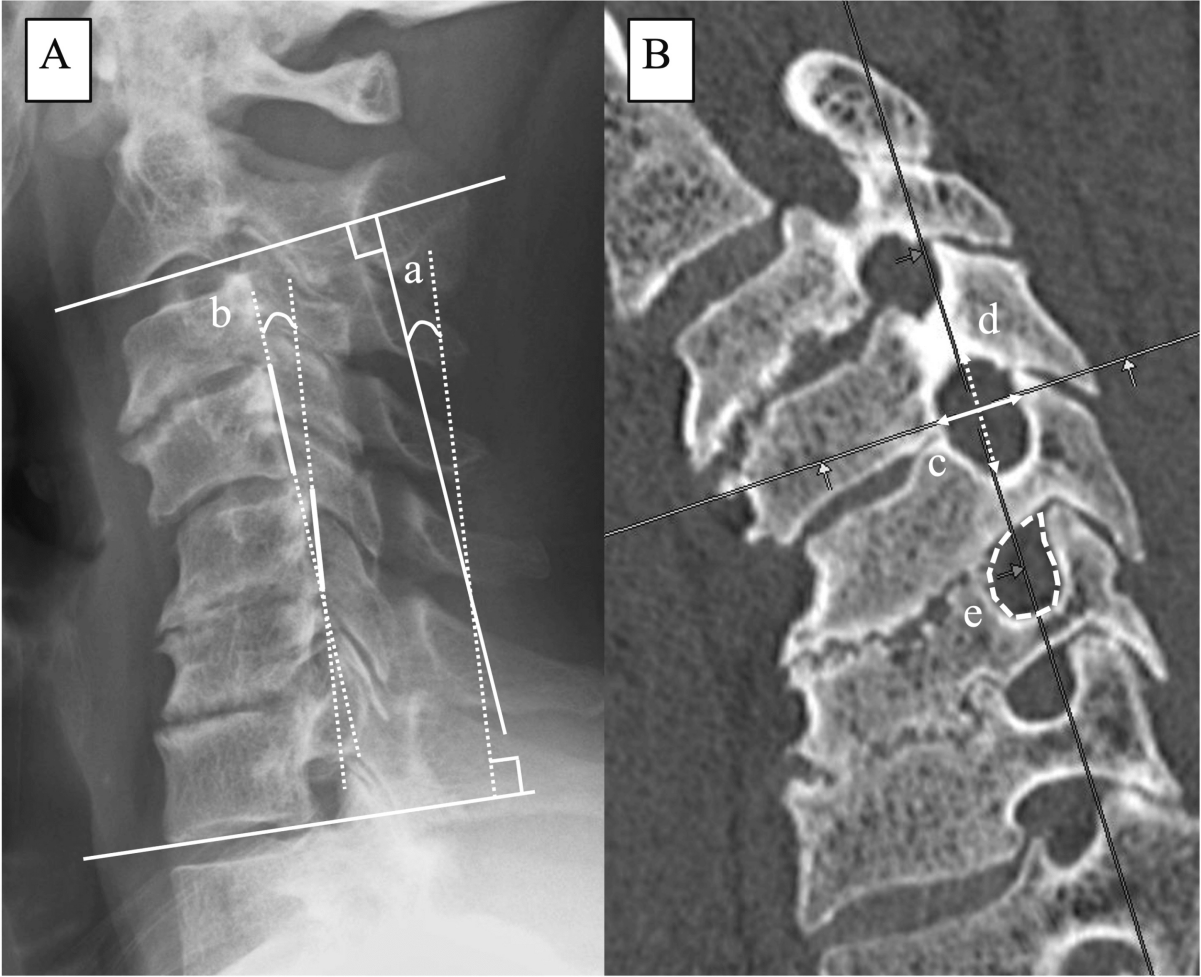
Radiological measurements and 3d-CT images. (A)  C2‒7 angle (a) and local segmental angle (b) were measured on a lateral radiograph in the neutral position. The segmental local angle (SA) was determined as the line from the posterior margins of the superior and inferior vertebrae to each targeted intervertebral foramen. (B) Sagittal CT image reconstructed using MPR.  c: The anteroposterior diameter (AP), d: craniocaudal diameter (CC), and e: foraminal area were measured using reconstructed 3D-CT images.
